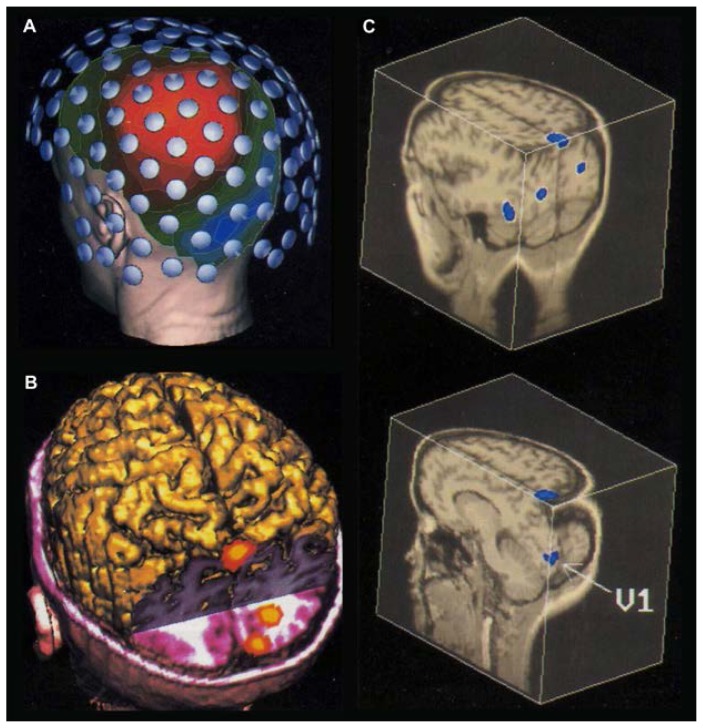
(A) Magnetoencephalogram evoked by a small visual stimulus in the subject’s lower right visual field. Magnetic field distributions (blue-red contours) are superimposed on a magnetic resonance-derived image of the subject’s head. Magnetic fields are displayed in red as they emerge from the head and in blue as they reenter. The white dots represent locations of the magnetic sensors. The data represent an average of 100 individual responses. (B and C) Vision-related areas of the brain’s outer layer (i.e., the cortex) are displayed by spatiotemporal analysis of the brain’s magnetic response to visual stimuli. Magnetic field sources are displayed in B as yellow-red contours and in C as blue contours. In B and C, magnetic field sources are displayed on images of the subject’s cortex derived from magnetic resonance imaging. Images courtesy of Doug Ranken.
